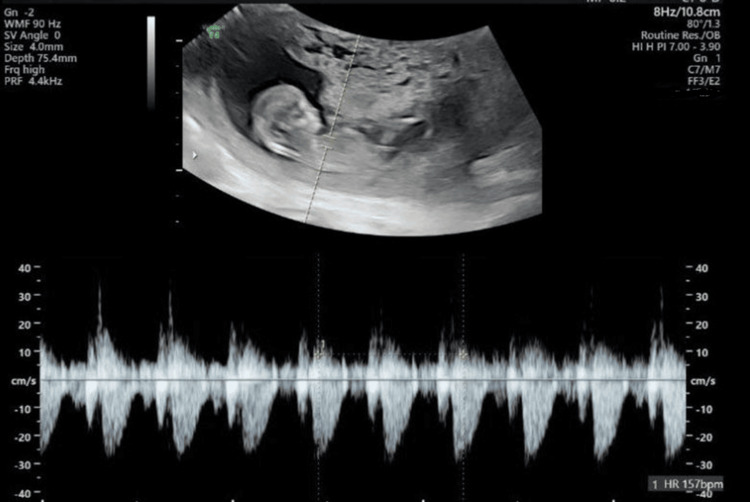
Ultrasound showing the viable co-twin (Twin A) with an appropriate crown–rump length and normal nuchal translucency at the end of the first trimester.
